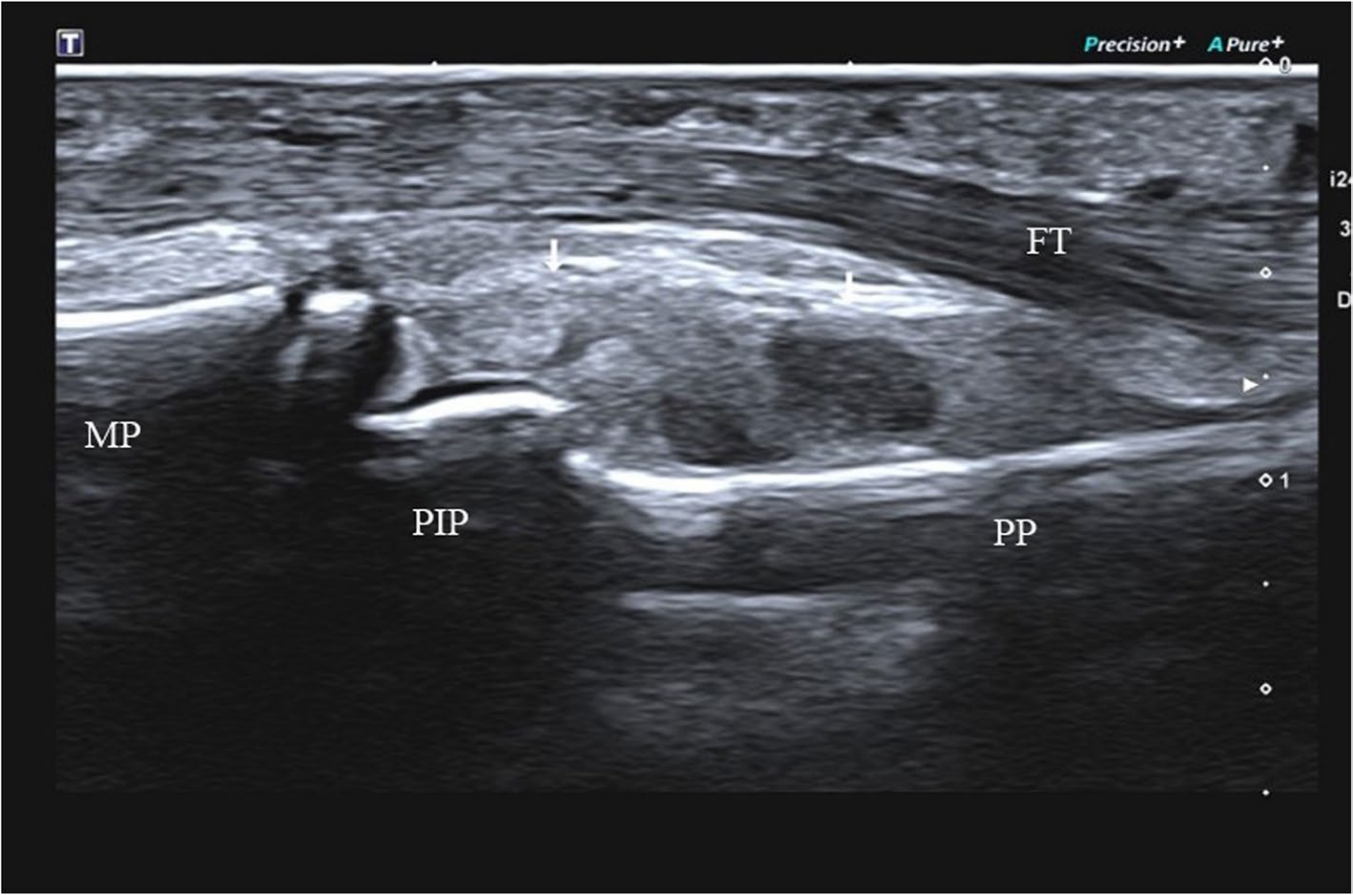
Type C (partial thickness tear of volar plate). High frequency ultrasonography image of type C volar plate injury of a 10-year-old boy in longitudinal plane. The ultrasonography showed the thicker and hypoechoic volar plate which was still integrate in longitudinal plane(arrow). MP: middle phalanx; PP: proximal phalanx; PIP: proximal interphalangeal joint; FT: flexor tendon
